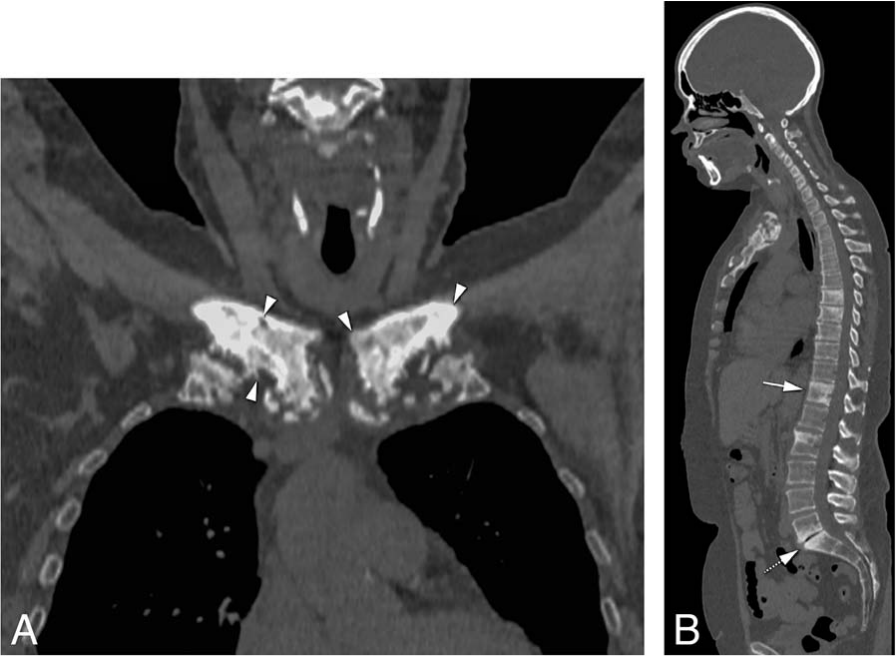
(A) Features of sclerosis, hyperostosis, and bone erosions of the medial end of clavicles and first ribs on coronal reconstruction of CT scan (arrowheads). (B) Sagittal image of CT scan showing characteristic patchy areas of sclerosis and mild hyperostosis of thoracic (T8, T9, TIO, T12) and lumbar (L2) vertebrae consistent with osteitis (arrow at T12 level). These features are different from reactive “kissing” vertebral plate sclerosis (Modic 3 changes) indicating degenerative changes associated with narrowing of the intervertebral space, intervertebral disk vacuum phenomenon, and absence of hyperostosis observed at L5/S1 (dashed arrow). The lesions were active on skeletal scintigraphy (not shown).
